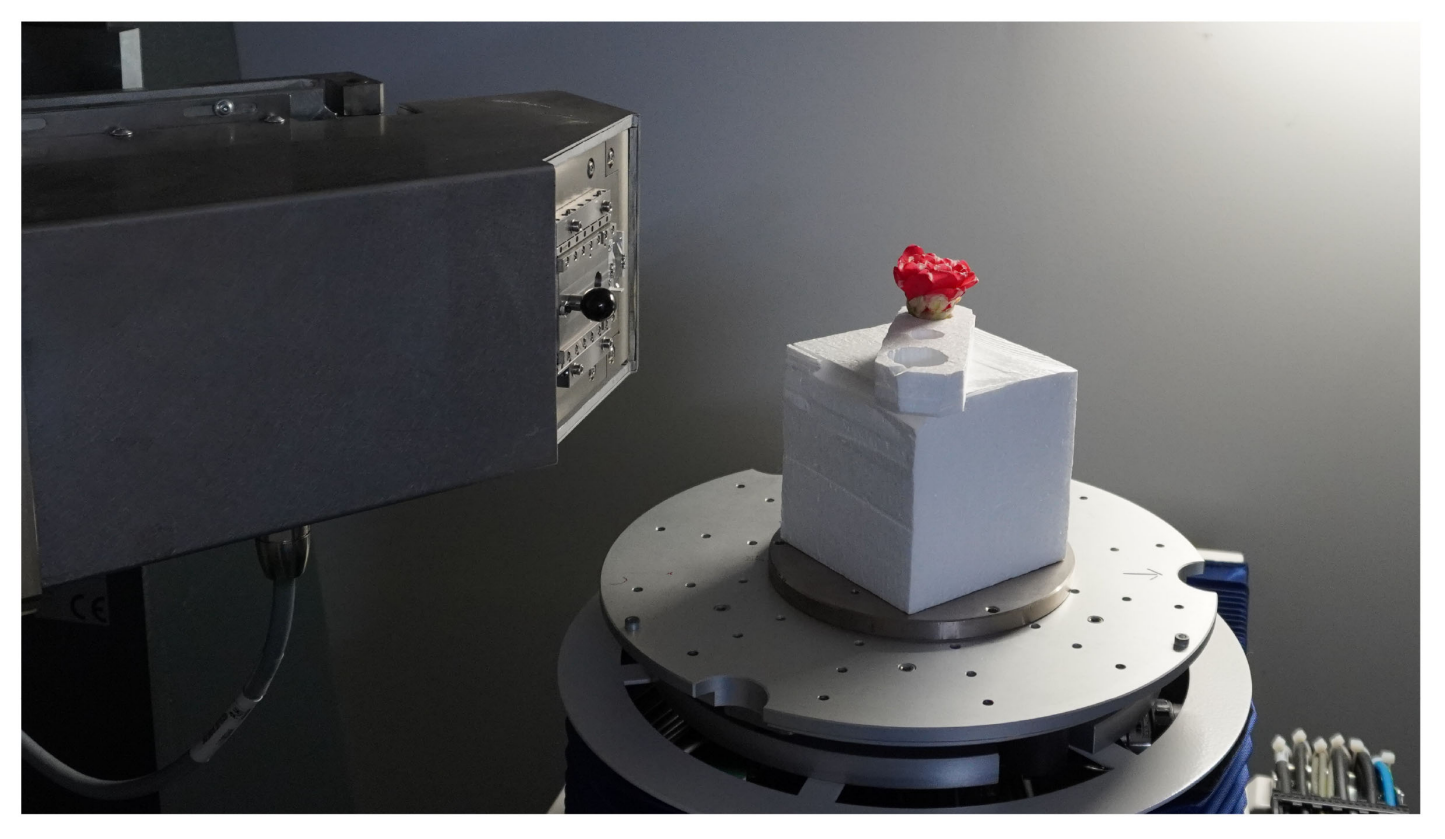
C. japonica* CT data acquisition. Image courtesy of Prof. Yutaka Ohtake, The University of Tokyo.
